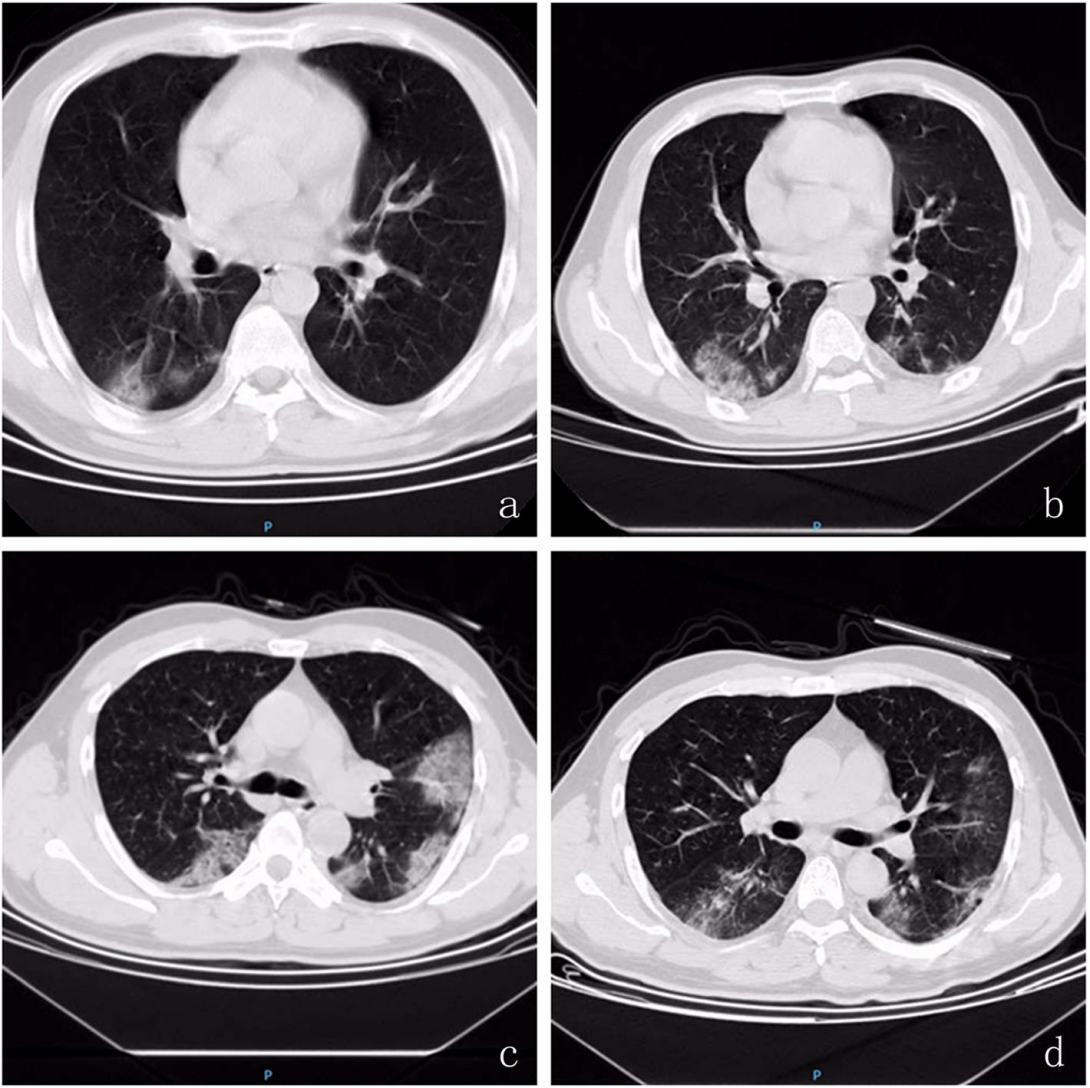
Chest CT scan image changes in COVID-19 Patients. At the day of confirm infection of 2019-nCoV, chest CT showed that multiple patchy sub-segmental or segmental ground-glass density shadow and consolidation were in bilateral lung (a severe cases & c mild cases); b Severe COVID-19 patient 5 days after confirm infection of 2019-nCoV, ground-glass density shadow and consolidation in bilateral lung was exacerbation; d Mild COVID-19 patient 5 days after confirm infection of 2019-nCoV, ground-glass density shadows in bilateral lung was absorbed
